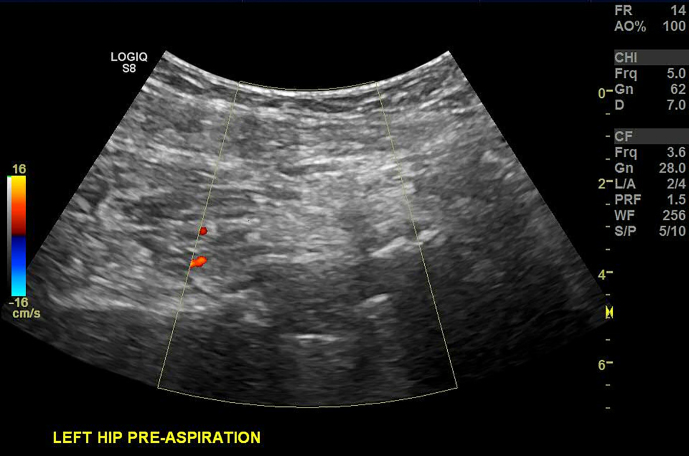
Ultrasound image of hip arthroplasty with color Doppler for
